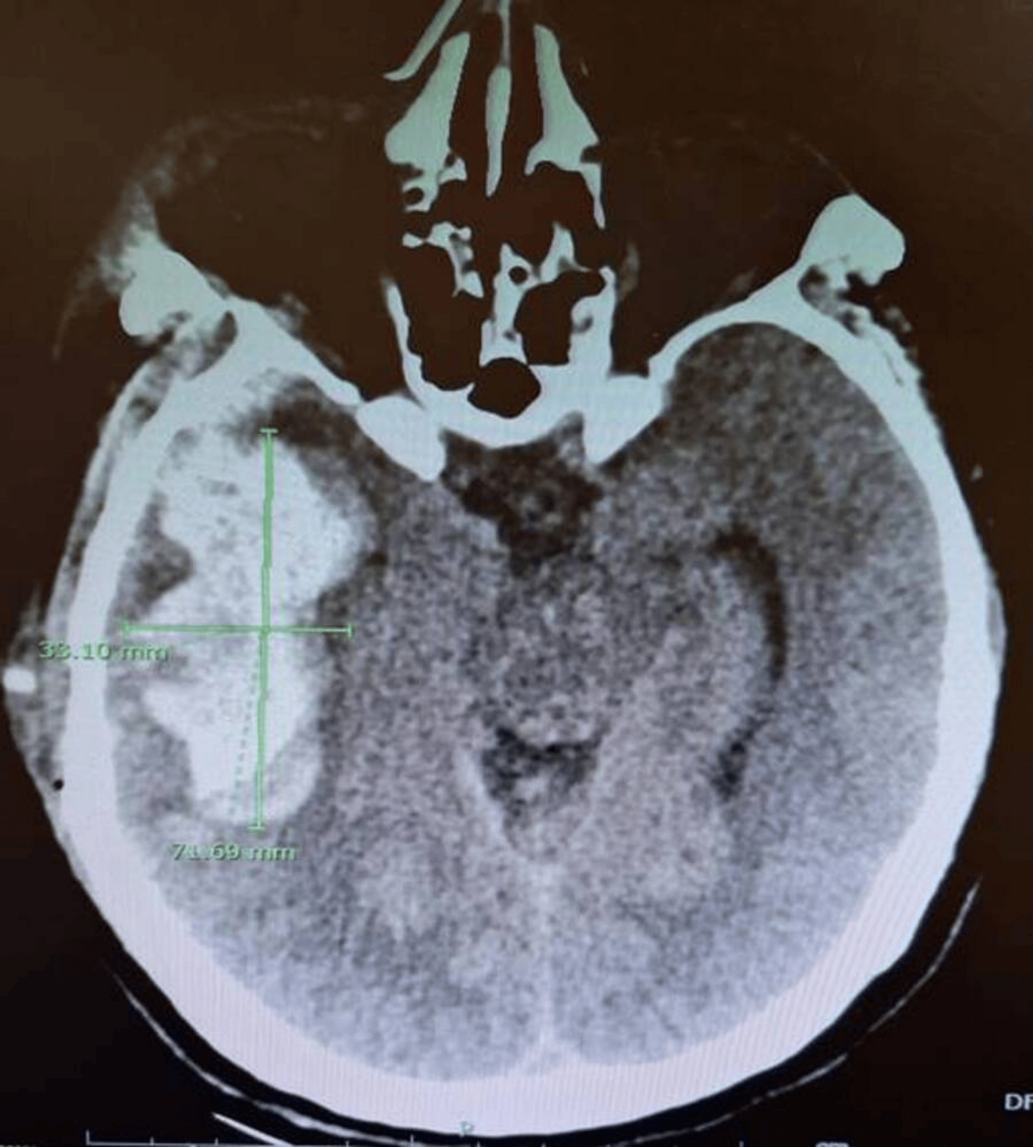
CT brain demonstrating large right parietotemporal hematoma.
